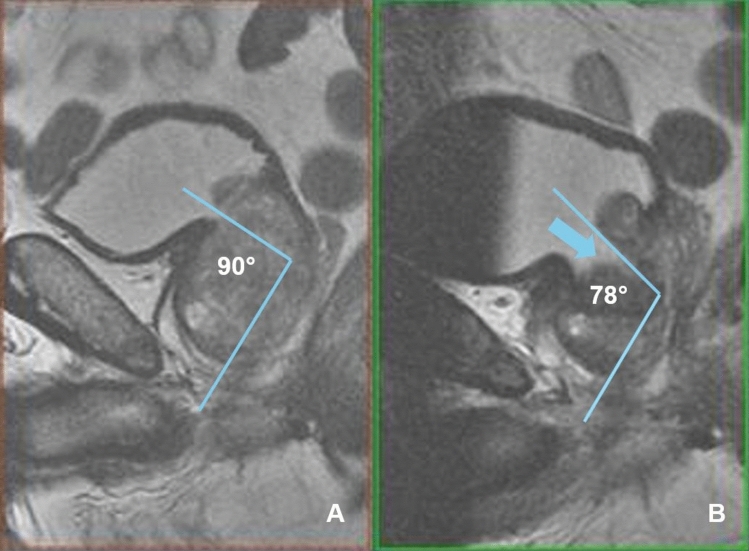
Sagittal T2-weighted image of the pelvis before (A) and 6 months after (B) prostatic cryoablation. In addition to the general reduction in prostate volume, successful opening and relief of the urinary bladder outlet can also be seen (arrow) with an improvement in the prostatic urethral angle
